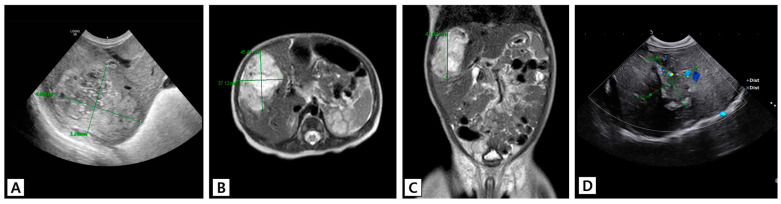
The US and MRI images of Patient 7, who had a large HH that partially resolved after treatment using a single therapeutic agent. (A) US performed on the day of birth shows a 4.8 × 3.3 cm sized mass on the right liver. The patient had mild feeding difficulty. (B,C) MRI performed on the ninth day after birth shows a large congenital hemangioma in the right lobe of the liver (S8 and S5) with internal necrosis and hemorrhage. Oral propranolol was initiated. (D) US performed at the age of 6 months shows that the largest diameter of the lesion decreased to 3 cm after treatment using oral propranolol. Abbreviations: HH, hepatic hemangioma; AFP, alpha-fetoprotein; US, ultrasound; MRI, magnetic resonance imaging.
